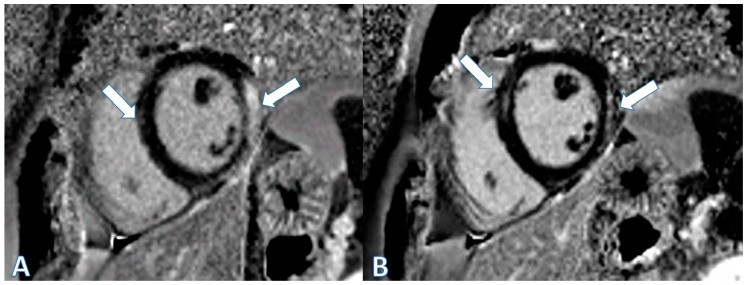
Cardiac MRI images. (A) Short-axis projection. Late enhancement sequence: subepicardial uptake affecting approximately 50% of the myocardial thickness in the lower-middle and inferolateral segments and the middle anteroseptal segment (arrows). (B). Short-axis projection. Follow-up at 2 years: persistence of LGE in the same locations, but with reduced extension (arrows).
